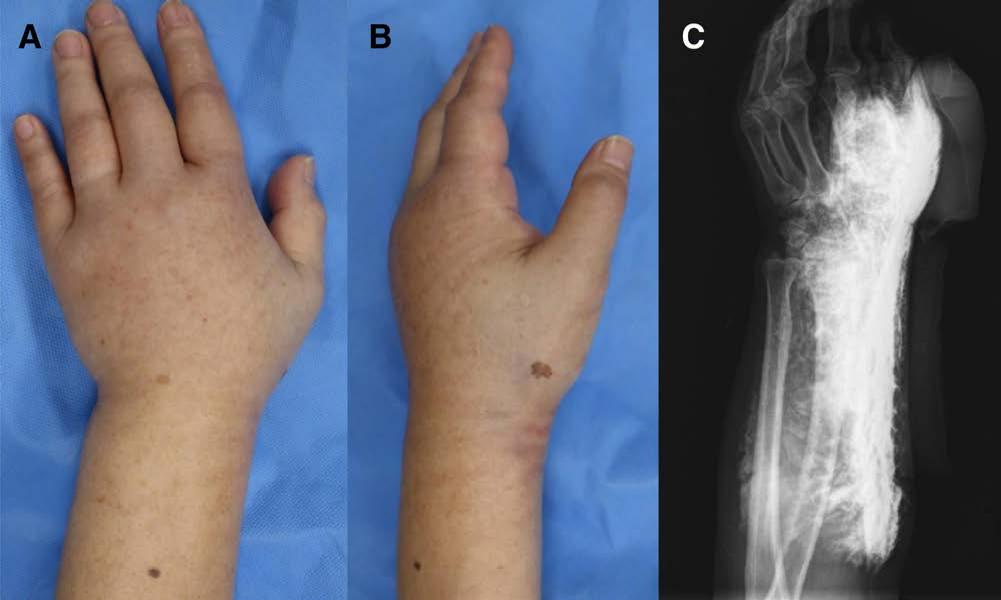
Initial evaluation findings of the reported patient’s left hand ~24 hours after contrast extravasation. (A) Dorsal view showing diffuse swelling with intact skin. (B) Lateral view confirming taut subcutaneous edema without visible color change or bullae. (C) Plain radiograph demonstrating diffuse soft tissue density compatible with extravasated contrast material; no gas formation or bony injury is observed.
